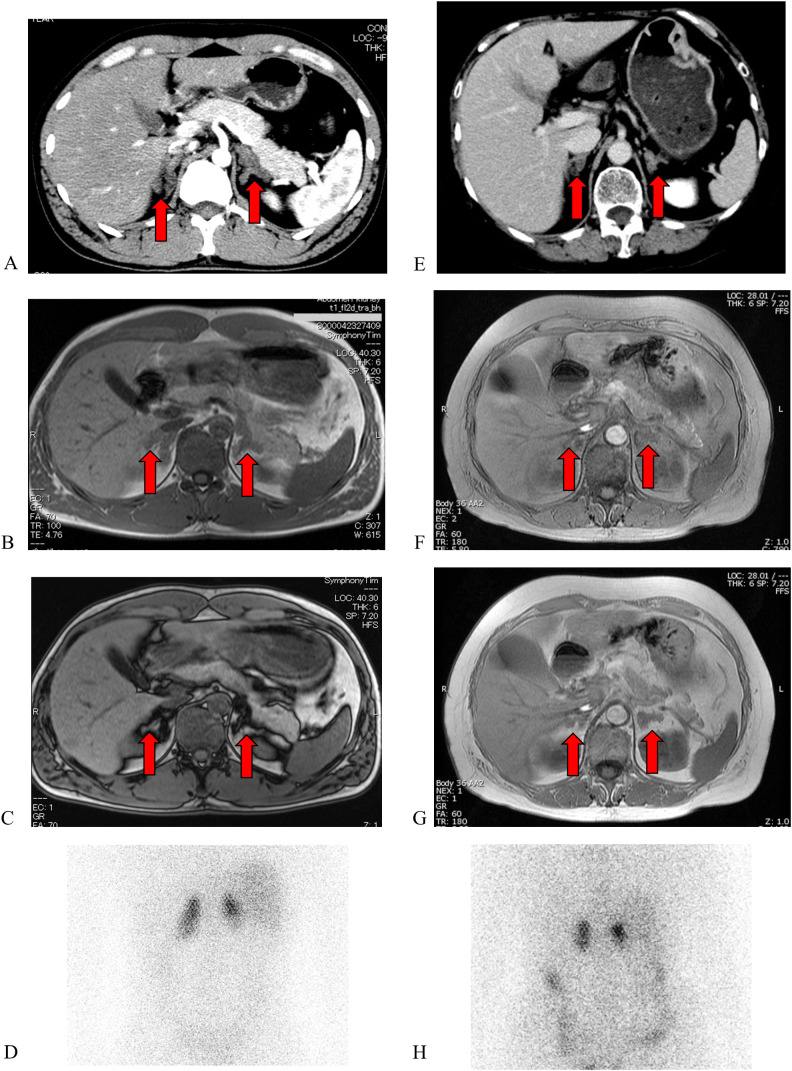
Various imaging findings of the adrenal glands in Patient 1 (A–D) and Patient 2 (E–H). (A, E) contrast-enhanced CT, (B, F) in phase magnetic resonance imaging (MRI), (C, G) opposed-phase MRI, (D, H)
